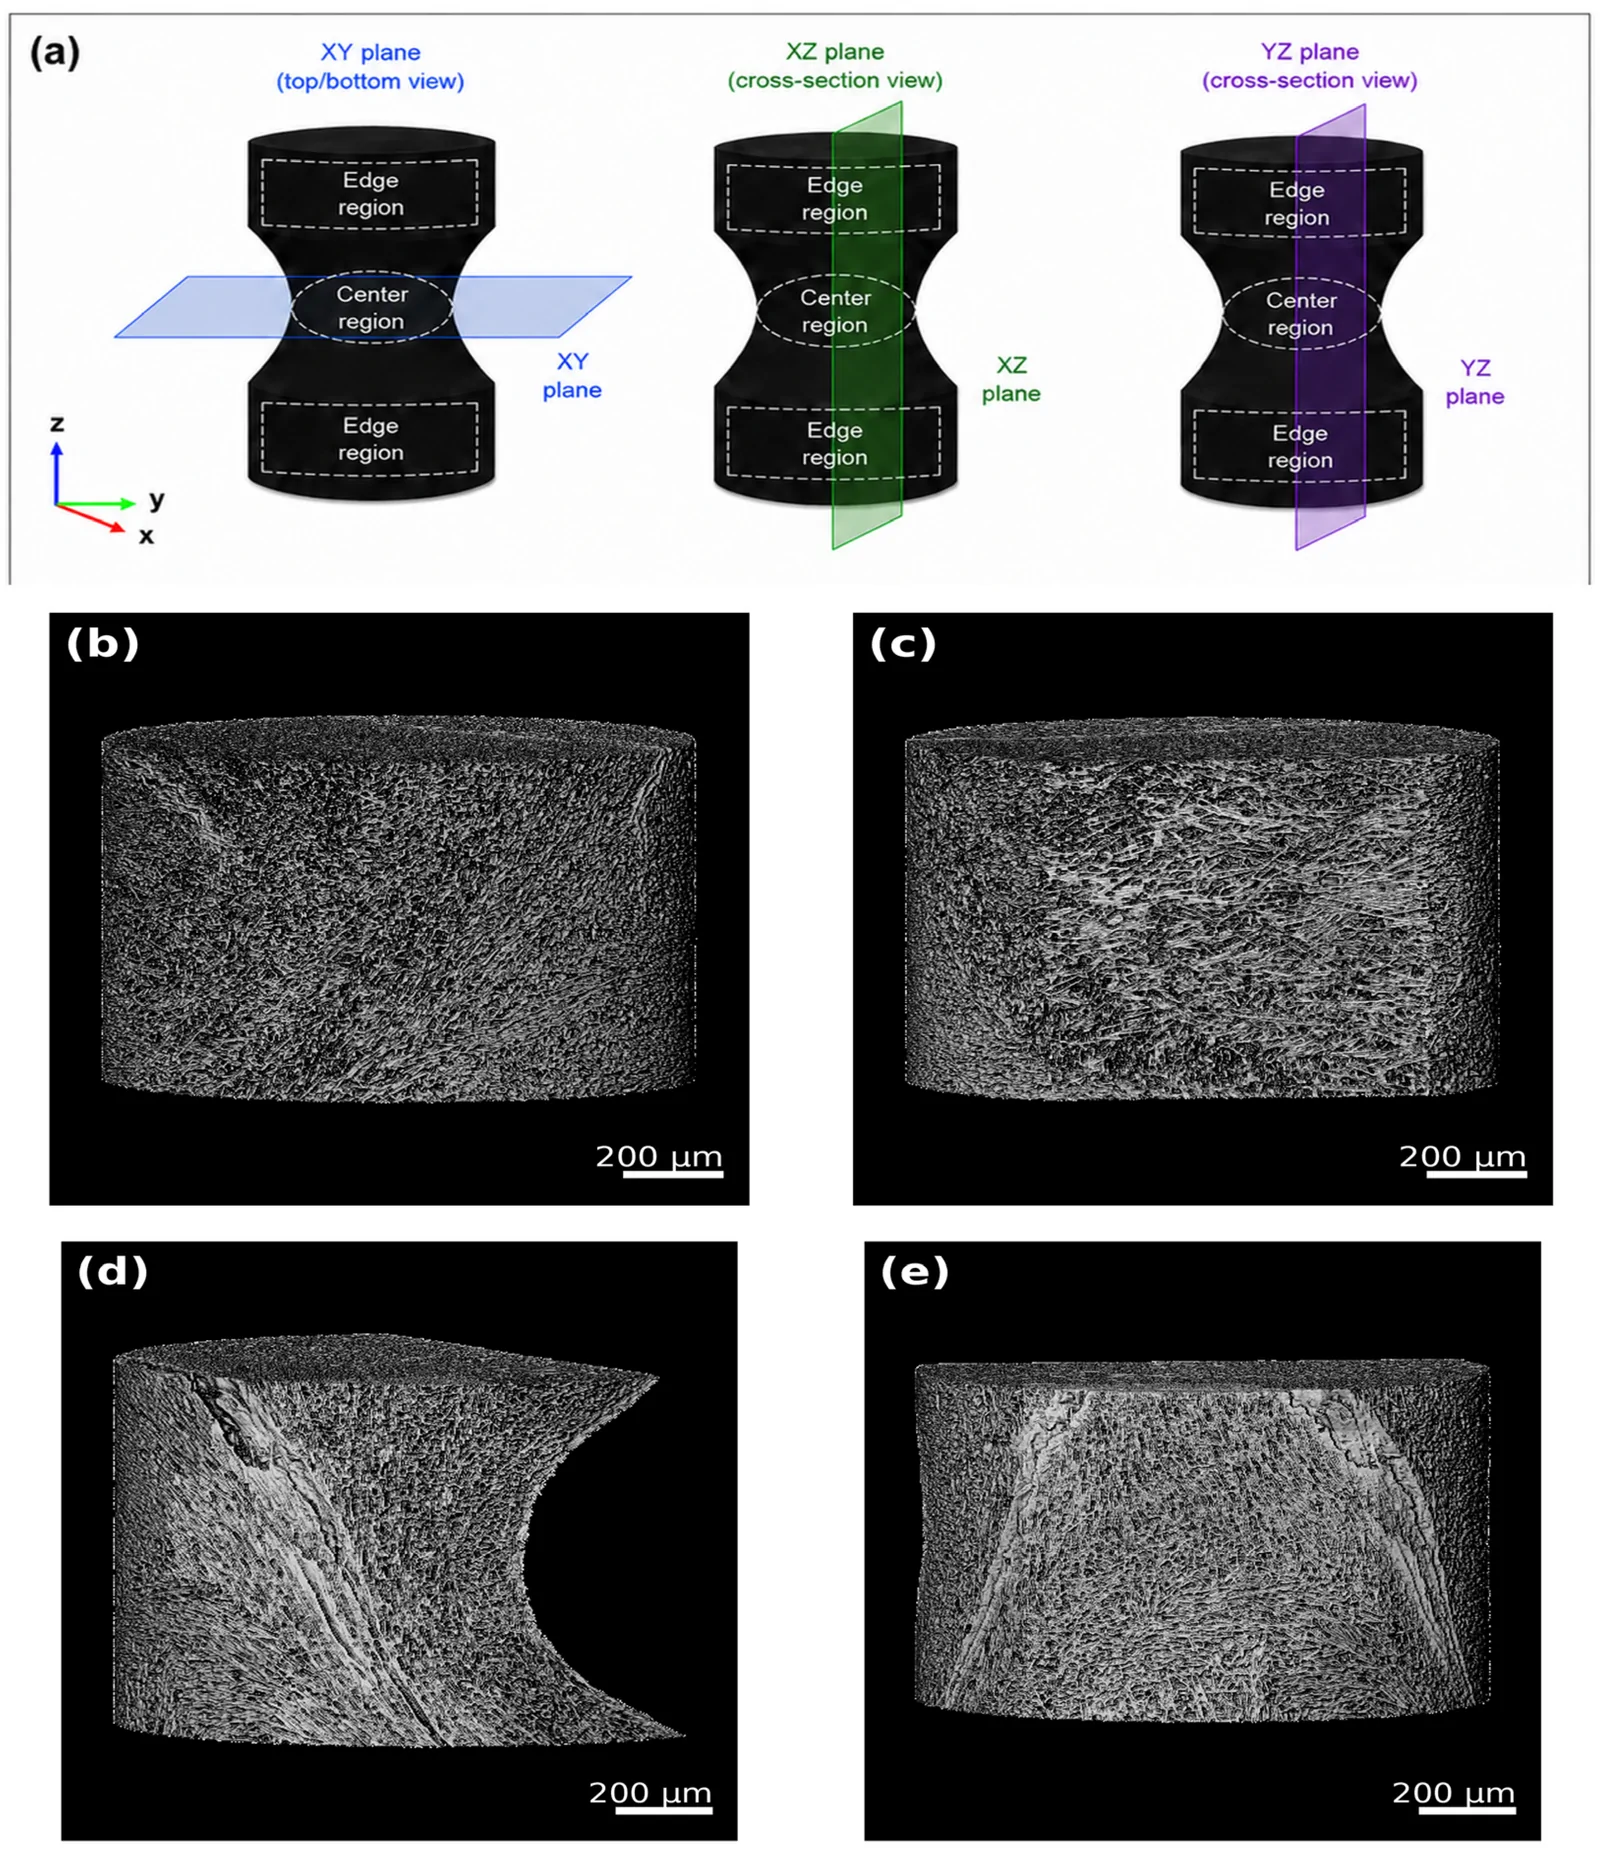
Synchrotron X-ray CT assessment of the web region between perforations in injection-molded PP/CF20/CNT20: (a) observation geometry and sectioning planes; (b,c) center region; (d,e) edge region. Scale bars = 200 µm.
